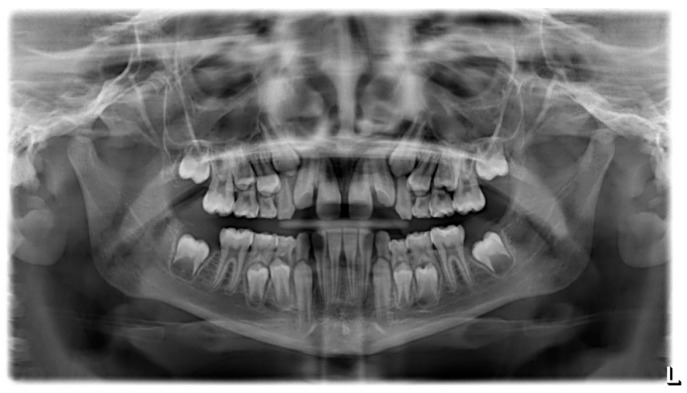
Orthopantomography X-ray after the treatment (clinical case #1).
